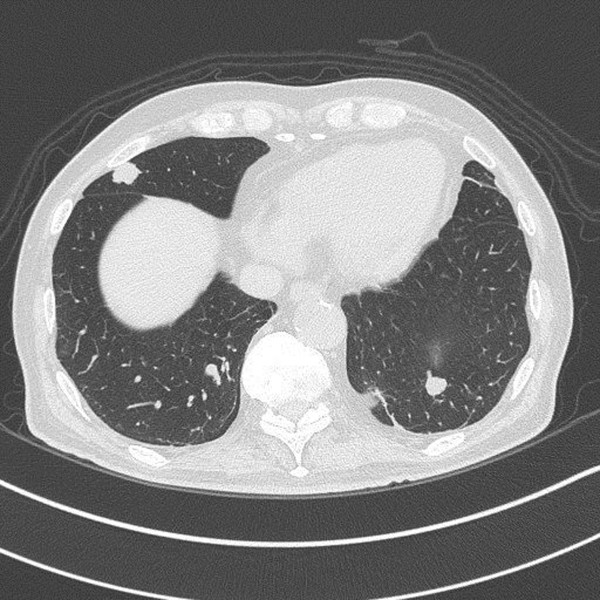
Chest computed tomography reveals multiple lung metastases, but the left atrium mass is unclear.
